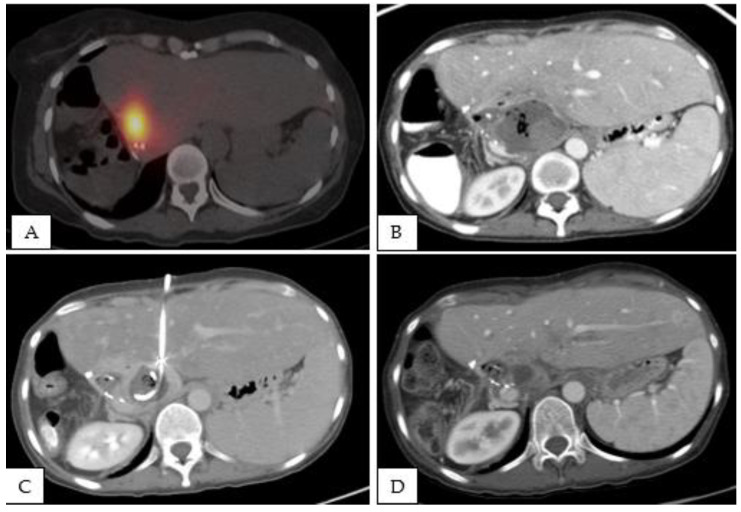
A 48-year-old woman with hepatocellular carcinoma and a history of hepaticojejunostomy received TARE. (A) SPECT CT following the ^90^Y treatment. The patient presented with worsening abdominal pain, fever, and mild leukocytosis 71 days after ^90^Y glass radioembolization. A contrast-enhanced CT scan of the abdomen and pelvis showed a 7.2 × 4.5 cm abscess (B). She was treated with intravenous antibiotics and a CT-guided percutaneous drain placement (C). The abscess drain was removed 31 days after discharge. After this, evaluation under fluoroscopy demonstrated that the small residual abscess cavity (D) was freely communicating with the biliary system and emptying through the hepaticojejunostomy.
